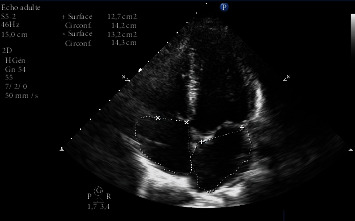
Transthoracic echocardiography of the mother.
